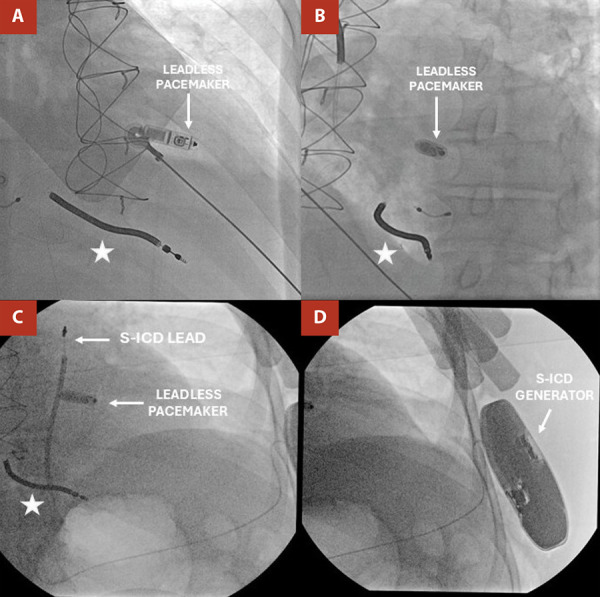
Leadless pacemaker implantation. (A, arrows) Fluoroscopy in right anterior oblique projection showing the leadless pacemaker implanted in the high mid-septal region of the RV. (B) Fluoroscopy in left anterior oblique projection confirms leadless pacemaker placement in the same septal region. S-ICD implantation. (C, arrows) Fluoroscopy image showing the S-ICD lead positioned at the third left parasternal ICS and the leadless pacemaker implanted in the mid-septal region of the RV. (D) Fluoroscopy image showing the S-ICD generator located at the 5th left ICS.
